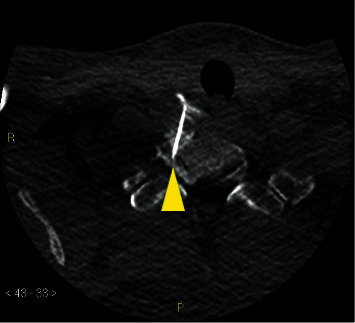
CT-guided injection. Axial section showing the tip of the 22G spinal needle at the right C7/T1 foramen. The contrast was injected to confirm flow centrally (yellow arrowhead).
